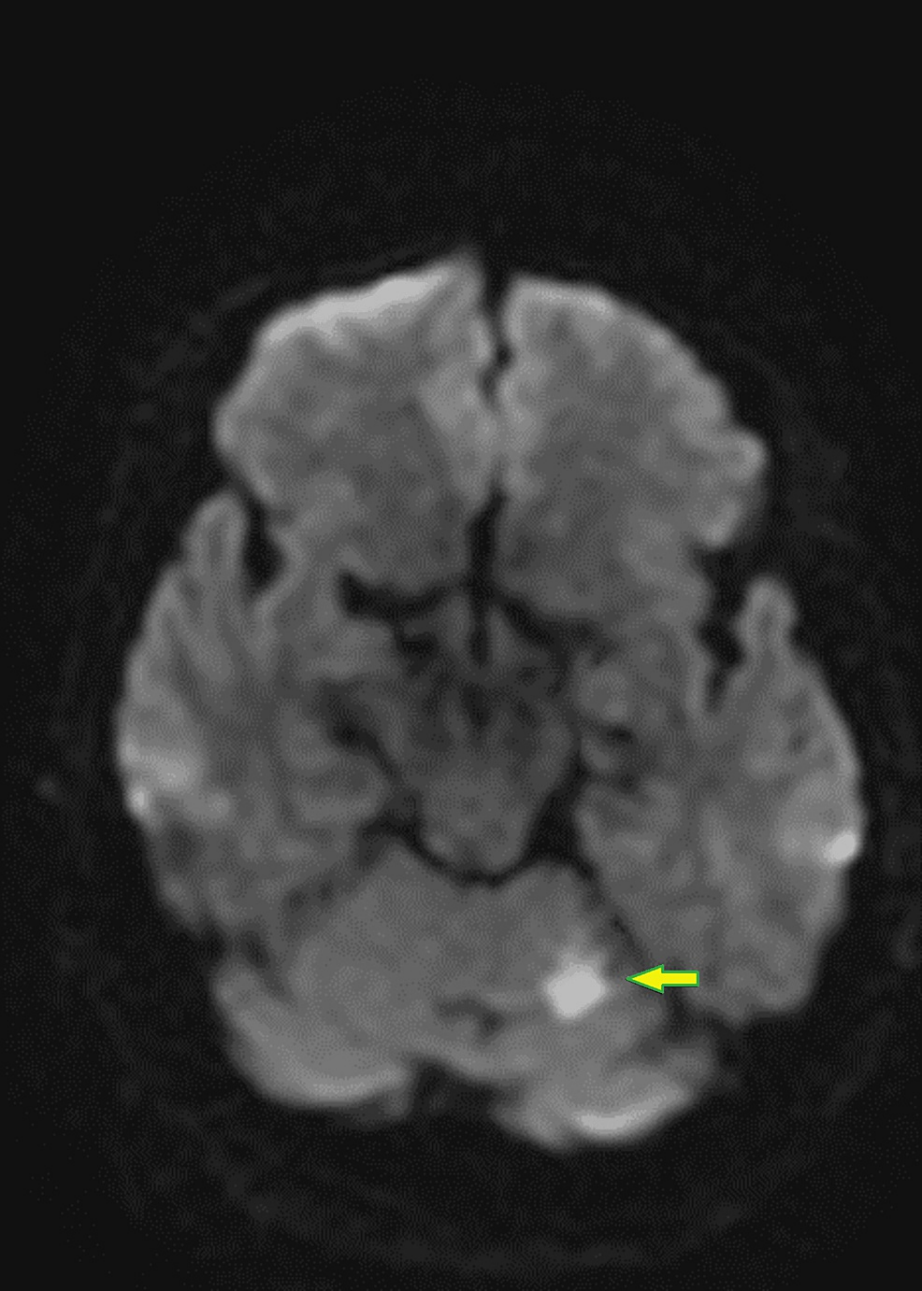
Brain MRI without contrast DWI sequence shows an acute appearing infarct in the left hemi-cerebellar hemisphere (arrow). MRI: magnetic resonance imaging, DWI: diffusion-weighted imaging
